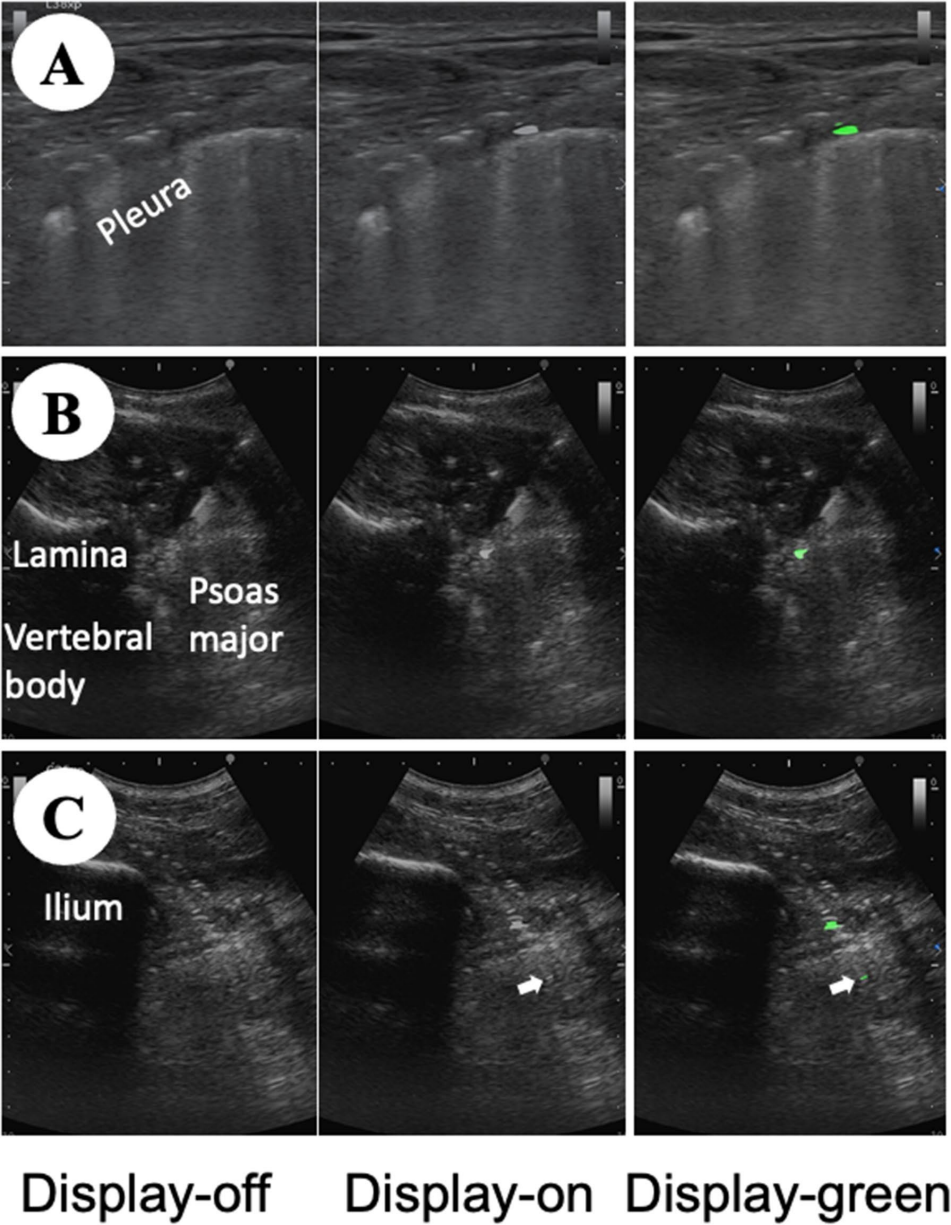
Ultrasound views of nerve blocks. The pictures show nerve blocks (at right side of cadaver). These pictures are all obtained using the photoacoustic needle. The picture of display-off does not show the photoacoustic ultrasound, the pictures of display-on and display-green show the photoacoustic ultrasound. Mirror image artifact of the needle tip is observed in the display-on and display-green of the (C) (white arrows indicate the mirror image artifact). (A) paravertebral block (A), (B) lumbar plexus block, (C) sciatic nerve block, display-off: no photoacoustic ultrasound, display-on: photoacoustic ultrasound using white color, display-green: photoacoustic ultrasound using green color (See Supplemental Digital Contents nos. 13, 15 and 17).
